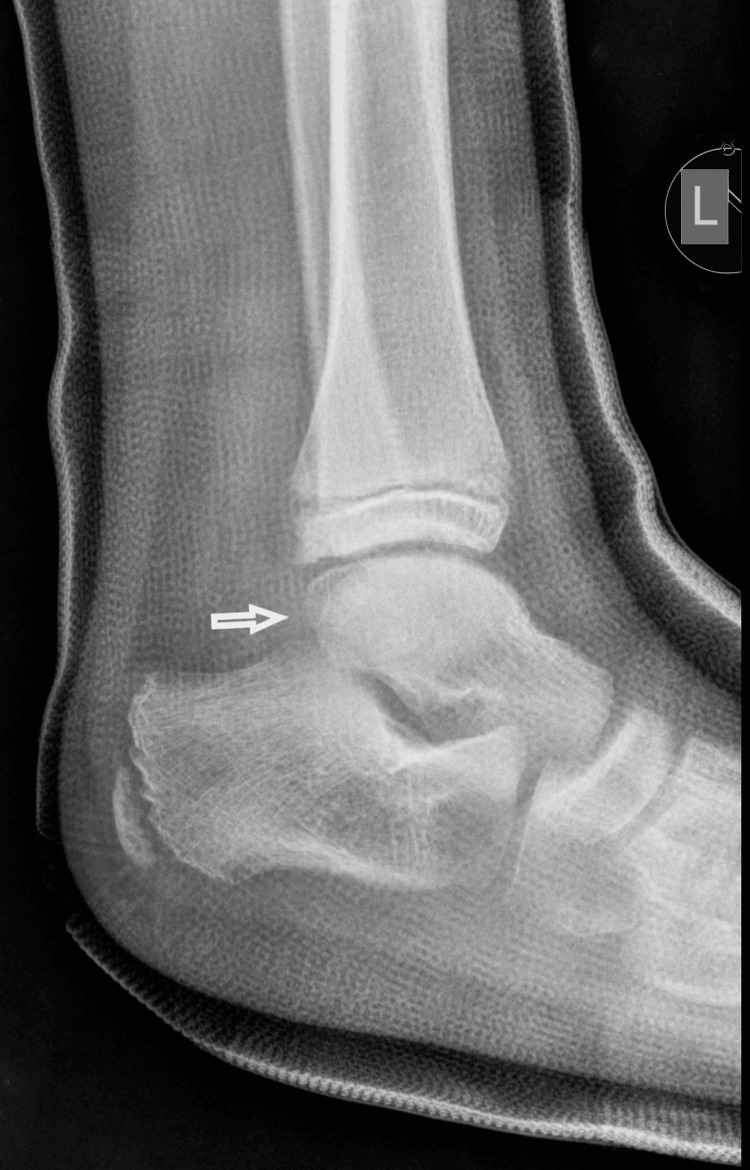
Lateral X-ray, after reduction of the fracture and immobilization in a cast. The arrow is pointing the normal alignment of the distal fibular epiphysis.
